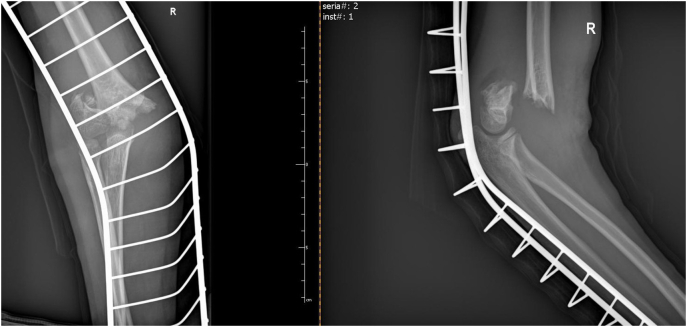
Preoperative radiograph of supracondylar humerus fracture. AP and lateral view.
